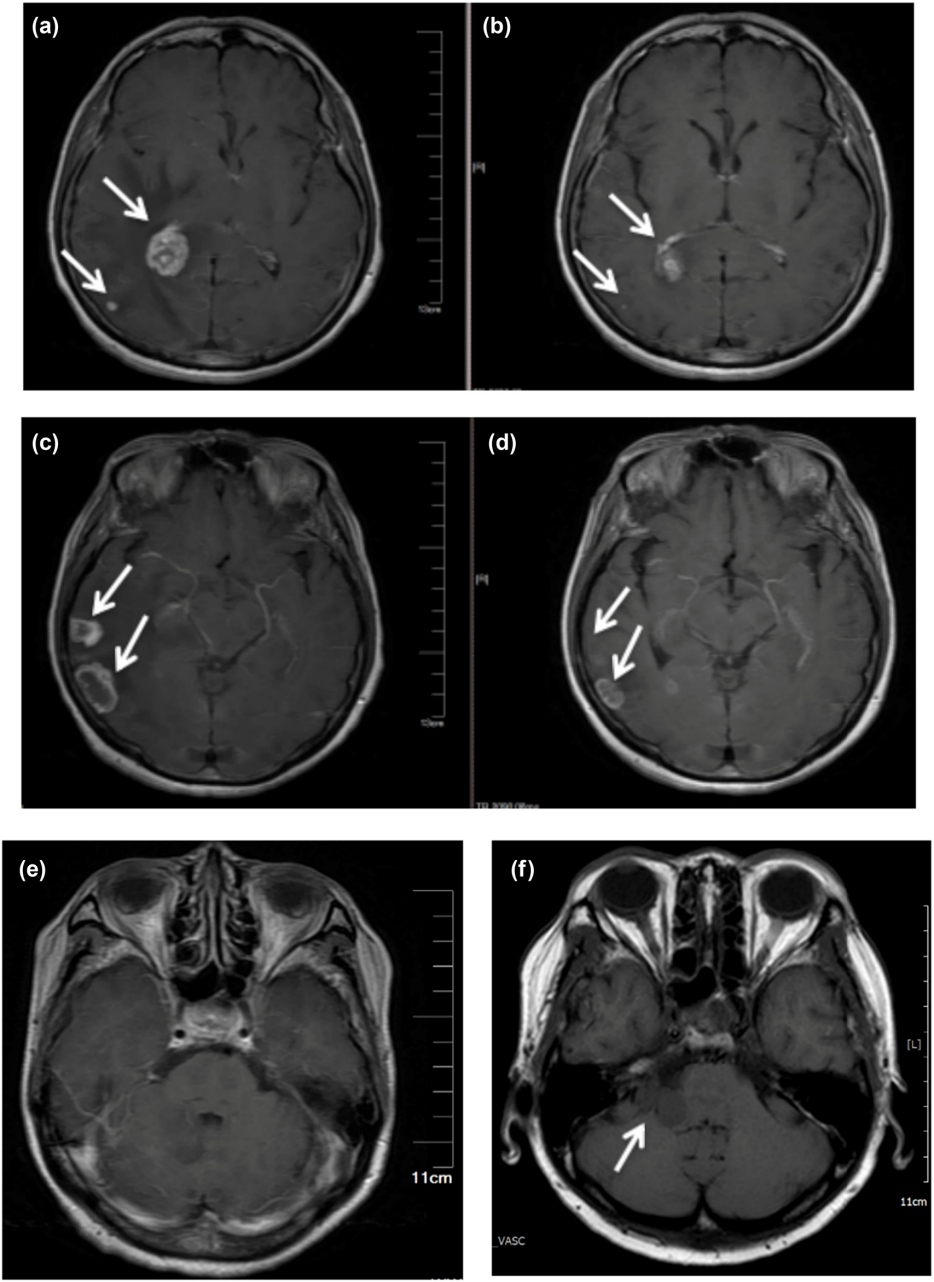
Multiple BMs revealed by MRI. (a and b), (c and d), and (e and f) are image comparison of the same part, respectively. (a), (c), and (e) At initial visit, (b) and (d) after targeted therapy of gefitinib, and (f) a new metastasis was found in the right cerebellar hemisphere.
